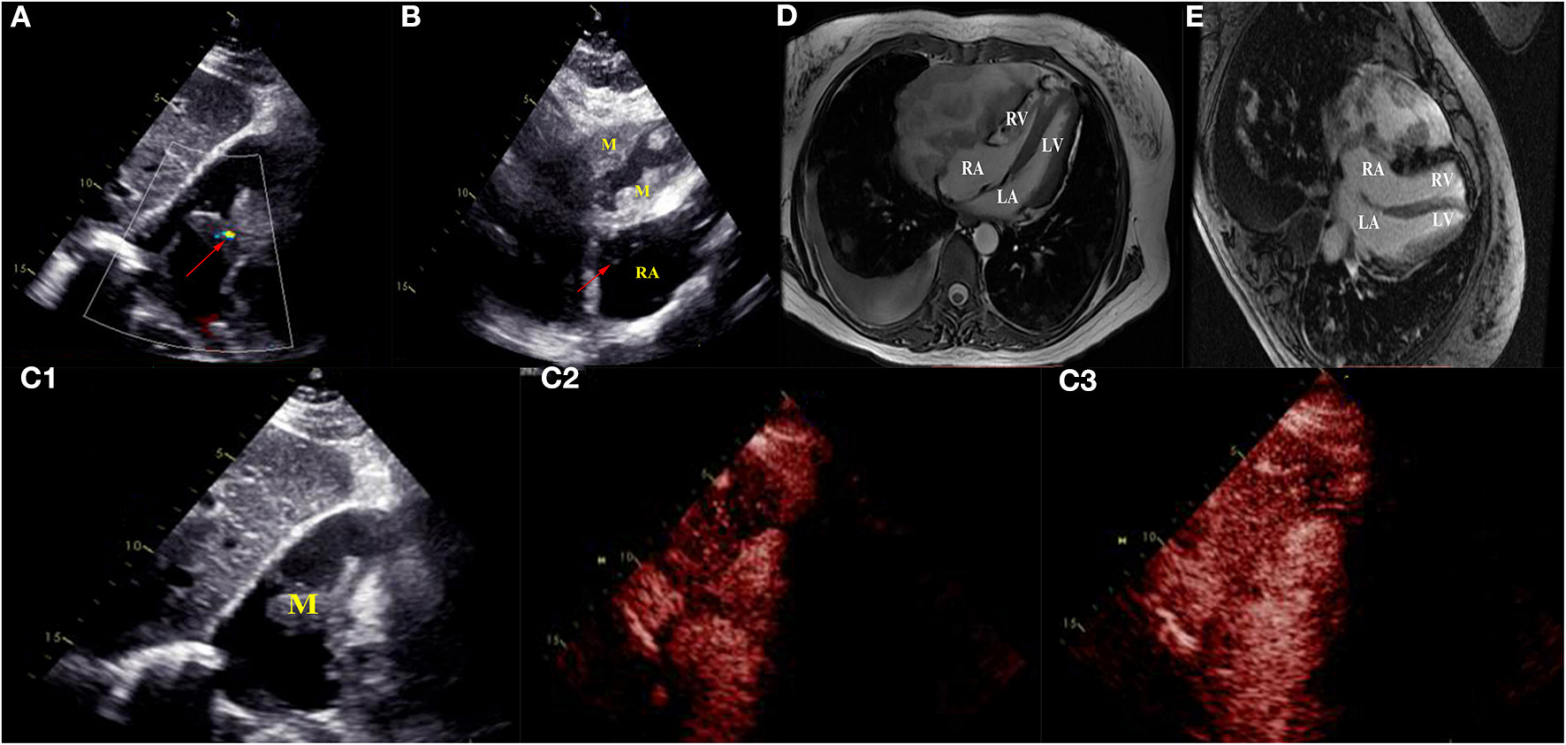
Transthoracic echocardiogram revealed a huge mass in the pericardium surrounding and compressing the right atrium and right ventricle (A,B). Mild blood flow signals (arrow) were seen in the mass (A) (May 20, 2020). The tumor was significantly larger than before and strip-like hyperechoism (arrow) inside the right atrium (B) (June 18, 2020). Contrast-enhanced ultrasound revealed the mass transferred right atrium, and the mass was rapidly filled with contrast agent (C1–C3) (May 20, 2020). Axial Fiesta (D) and 4CH-2D (E) images of cardiac magnetic resonance showed an irregular, large tumor (size up to 10 × 5.5 cm) with heterogeneous distinction diffusely within the pericardium (10 × 5.5 cm), mainly locating outside of right cardiac system, and compressed the right heart. RA, right atrium; RV, right ventricle; LA, left atrium; LV, left ventricle; M, mass; RA, right atrium.
